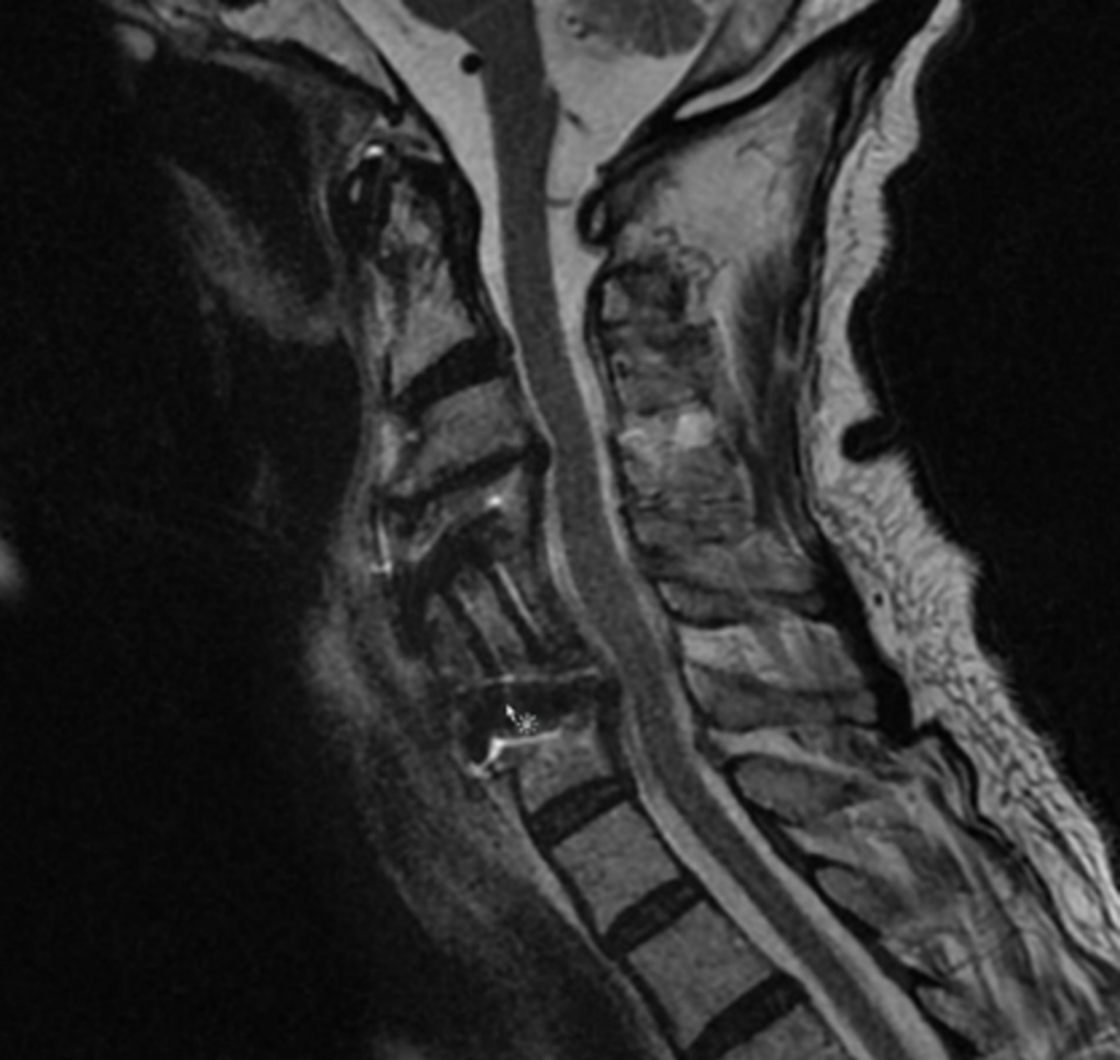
Postoperative T2-weighted cervical spine MRI in the sagittal plane showing expected postoperative changes with no residual compression of the dural sac
